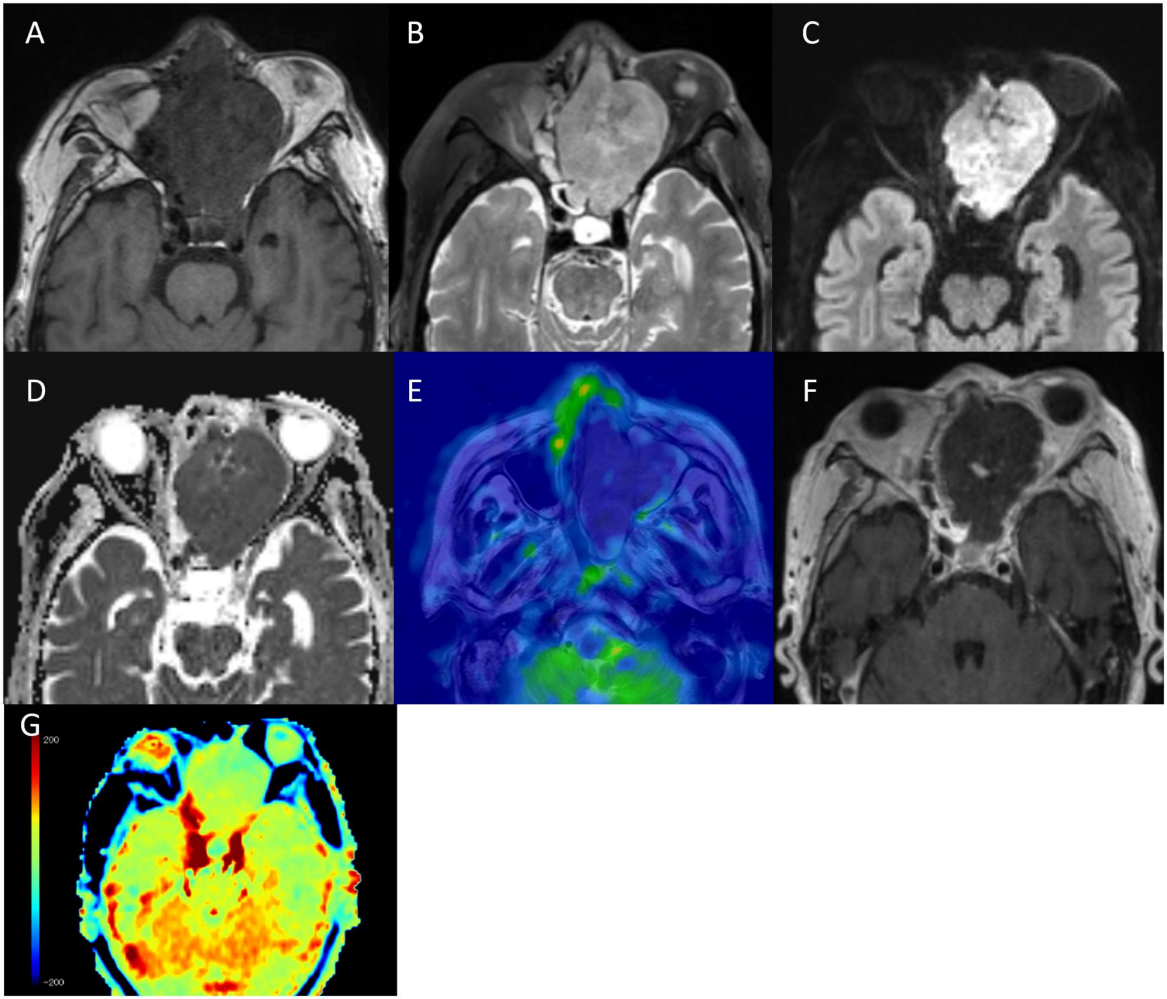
(A-G) MRI on day 16 of admission. The tumour in the left ethmoid sinus shows an isointense signal in comparison to muscle on T1WI (A), a hyperintense signal on T2WI (B), and a hyperintense signal with a low ADC value (mean ADC value 0.72 × 10^−3^ mm^2^/s) on DWI (C, D). Arterial spin label perfusion-weighted imaging (ASL-PWI) shows a hypointense signal in the tumour (E). Gd-T1WI shows an extensive unenhanced area in the tumour (F), suggesting spontaneous infarction of the tumour (E, F). APT-CEST imaging shows a slight hypointense signal in the unenhanced area within the tumour in comparison to normal brain parenchyma (G).
